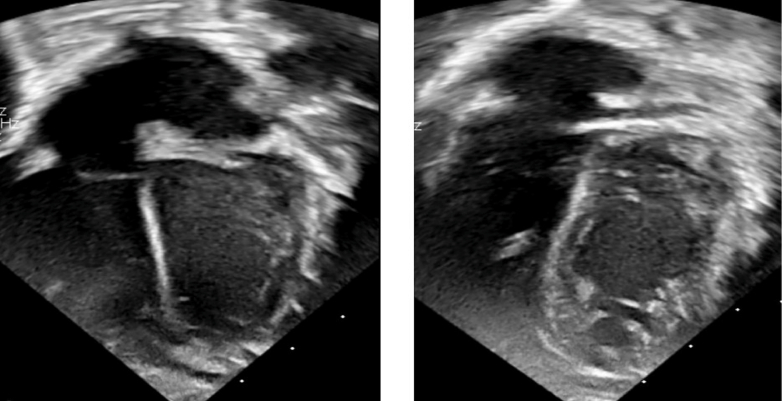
Control vs Noncompaction Echocardiogram Echocardiogram (apical 4-chamber view) depicting normal cardiac anatomy in age-matched control (left) vs noncompaction cardiomyopathy in patient (right).
